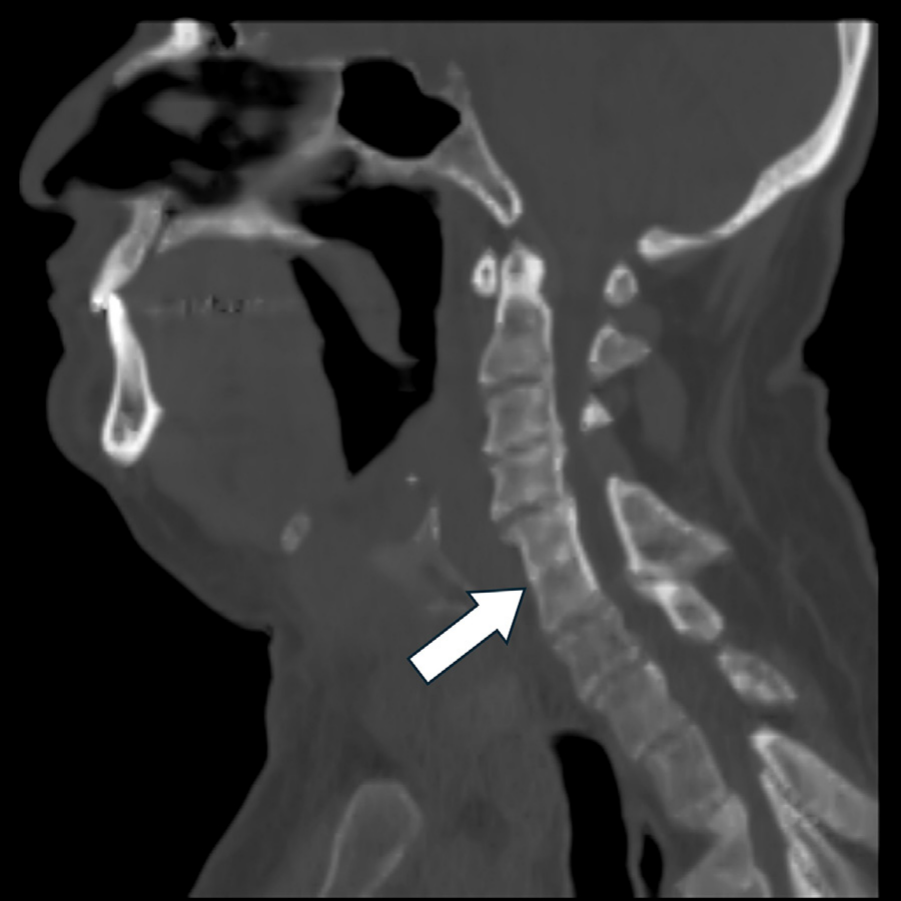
Sagittal CT shows fusion of C5 and C6 vertebrae, including the vertebral bodies (white arrow) and posterior elements. There is narrowing of the anteroposterior dimension of the C5 and C6 vertebral bodies. These findings are consistent with Klippel-Feil syndrome. No spinal hardware is seen to indicate prior surgery.
